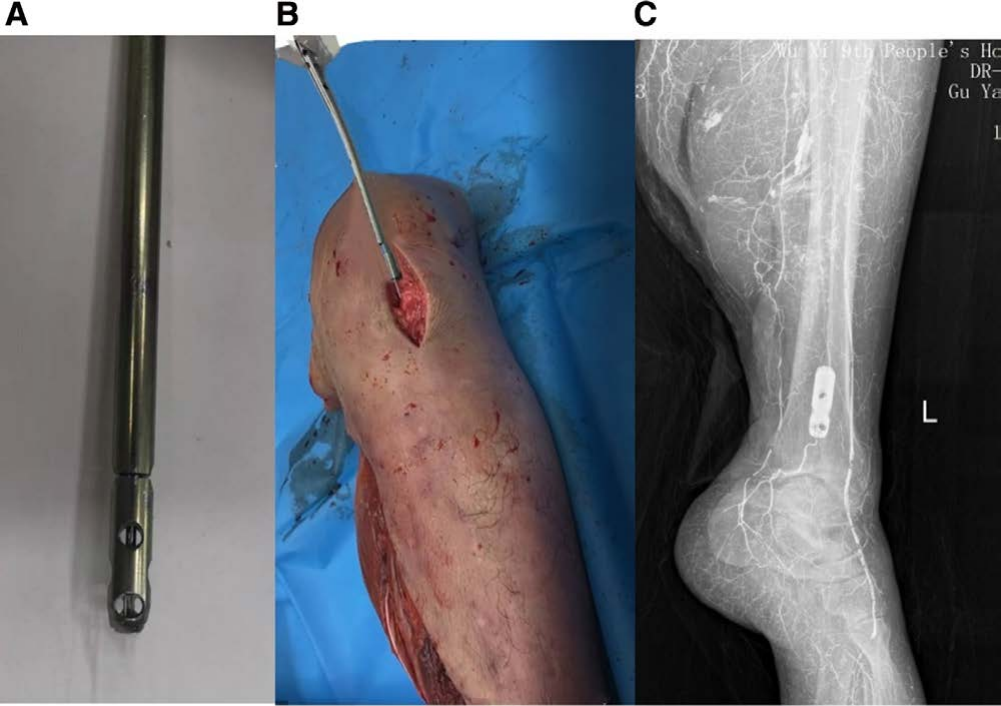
Establishment of the tibial broken nail model. (A) Breaking of the distal tibial intramedullary nail. (B) The broken front was placed in the medullary cavity of the distal tibia under the guidance of the guide pin. (C) X-ray showing the broken nail located in the medullary cavity of the distal tibia.
